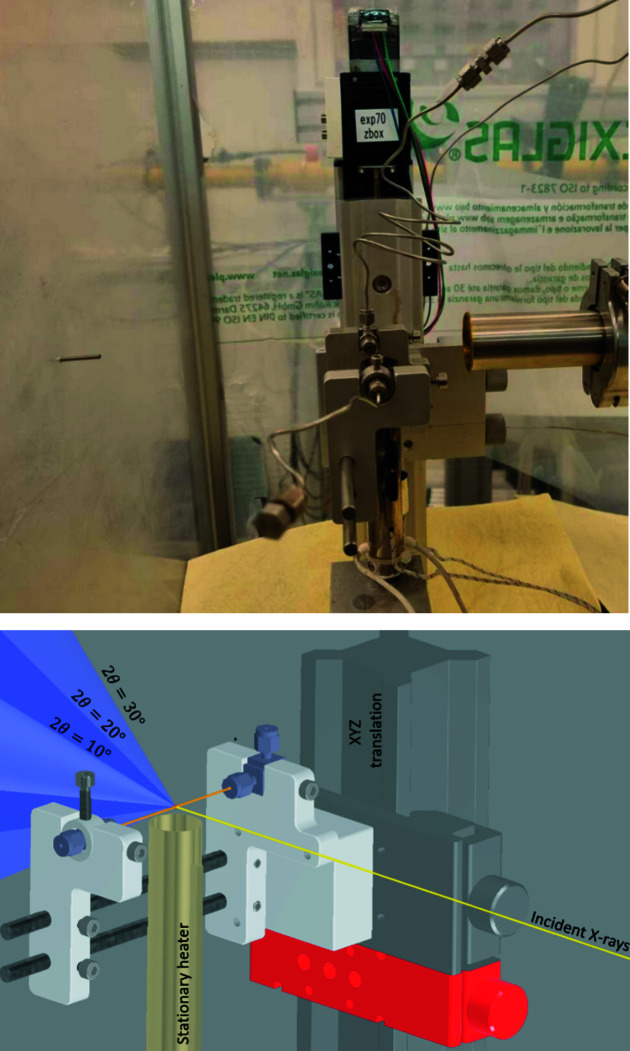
(Top) Photograph of the cell installed on the P21.1 beamline. (Bottom) A CAD rendering with the heater assembly centred 6 mm below the sample. The incident X-ray beam is depicted as a yellow line, with Debye–Scherrer cones from the capillary at 10, 20 and 30° in 2θ.
